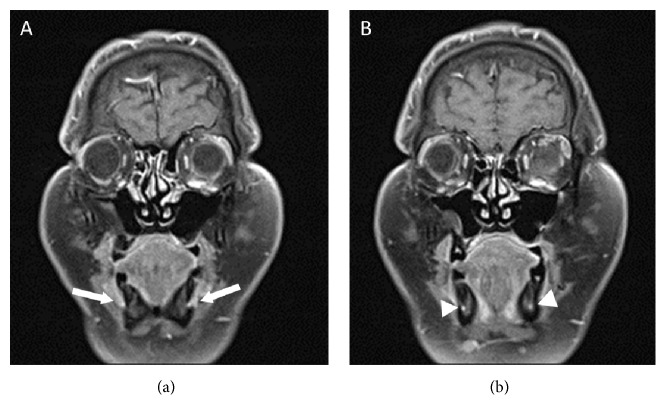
Serial coronal contrast-enhanced T1-weighted images of the face showing perineural infiltration with thickening and enhancement of the mental nerves bilaterally (arrows) with involvement of the inferior alveolar nerves as well (arrowheads).
